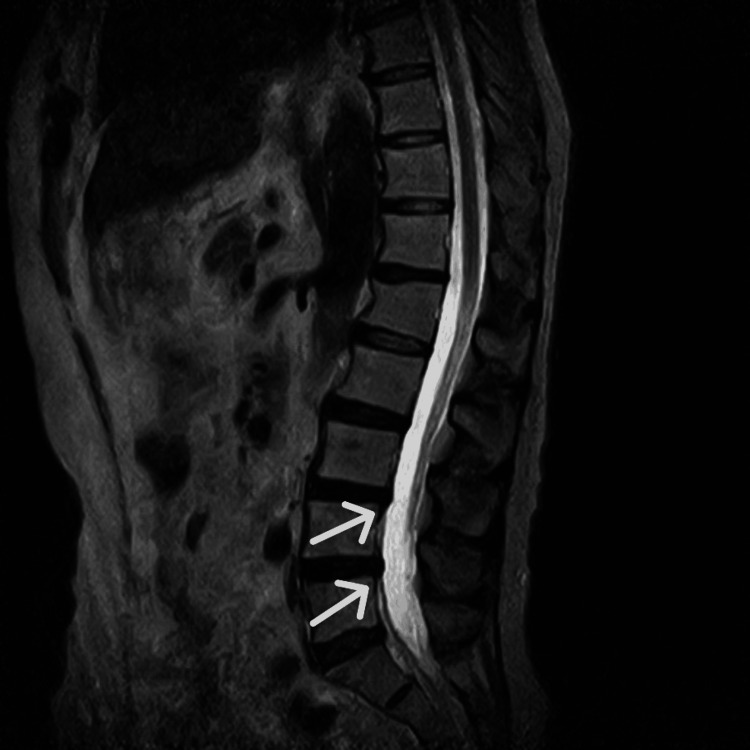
Sagittal T2-weighted MRI showing degenerative lumbar spondylosis and disc bulging at the L3/4 and L4/5 levels
